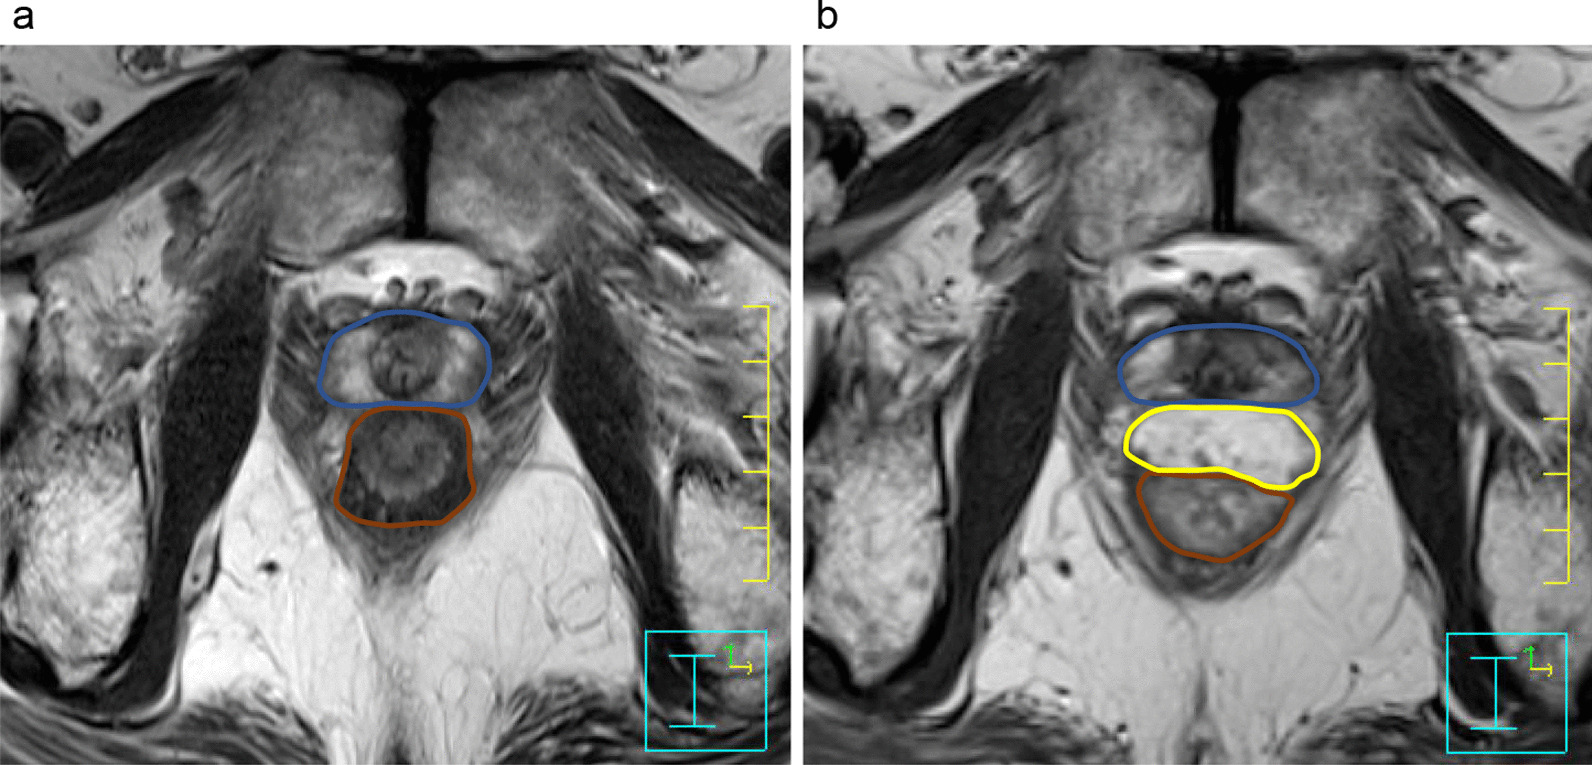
MRI images of the same patient. a The image before spacer injection. b The image after spacer injection. Prostate (blue), rectum (brown), and spacer (yellow) are shown
